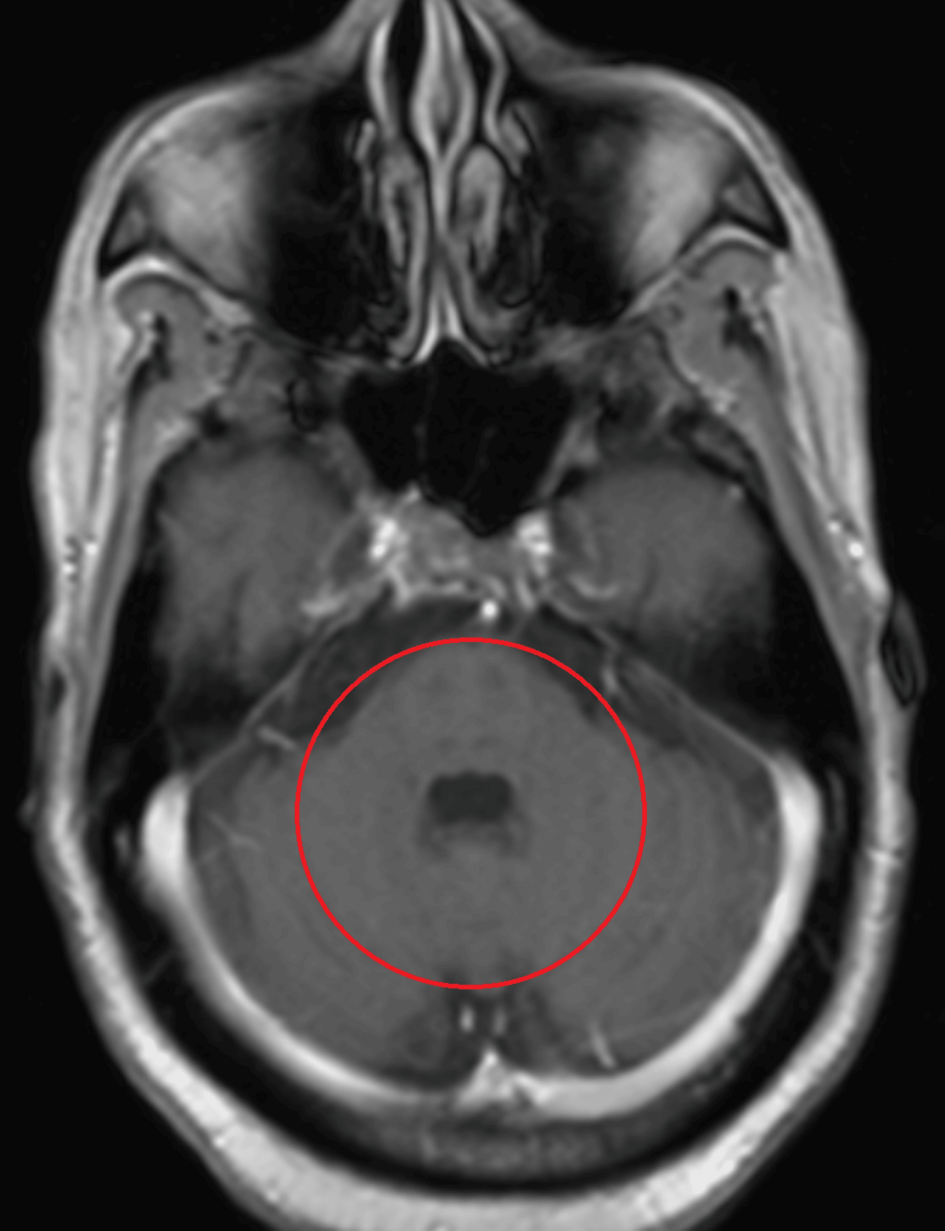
T1 postcontrast image of brain MRI showing no enhancing lesion in pons or cerebellum. The red circle shows the area of interest.
